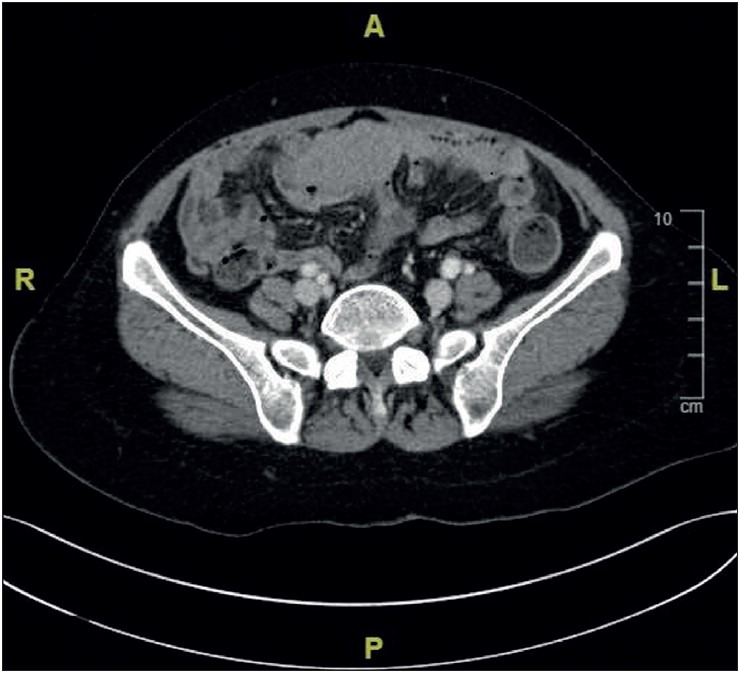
Axial computed tomography images of the pelvis.
